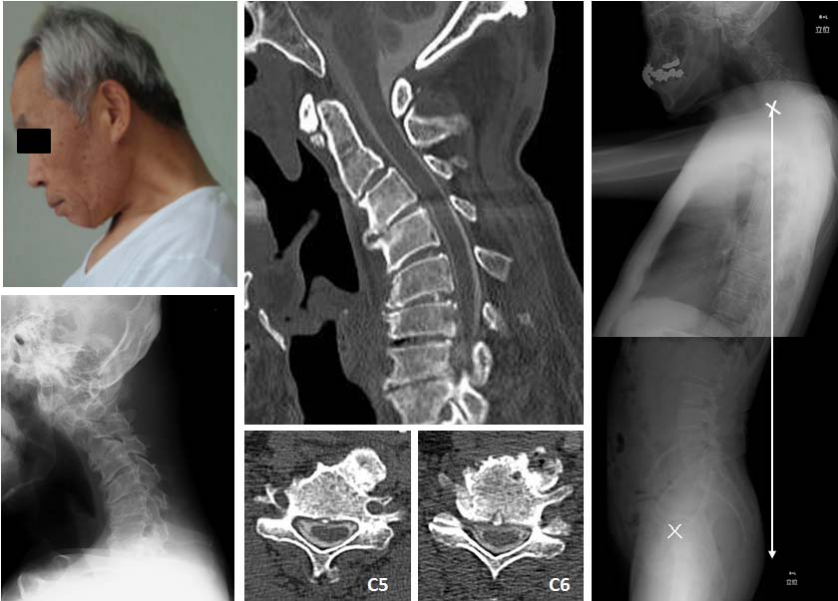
Dropped head deformity before surgery. Radiographs showing degenerative kyphoscoliosis with ossification of the anterior longitudinal ligament. Impairment in total spinal alignment due to cervical kyphosis.
